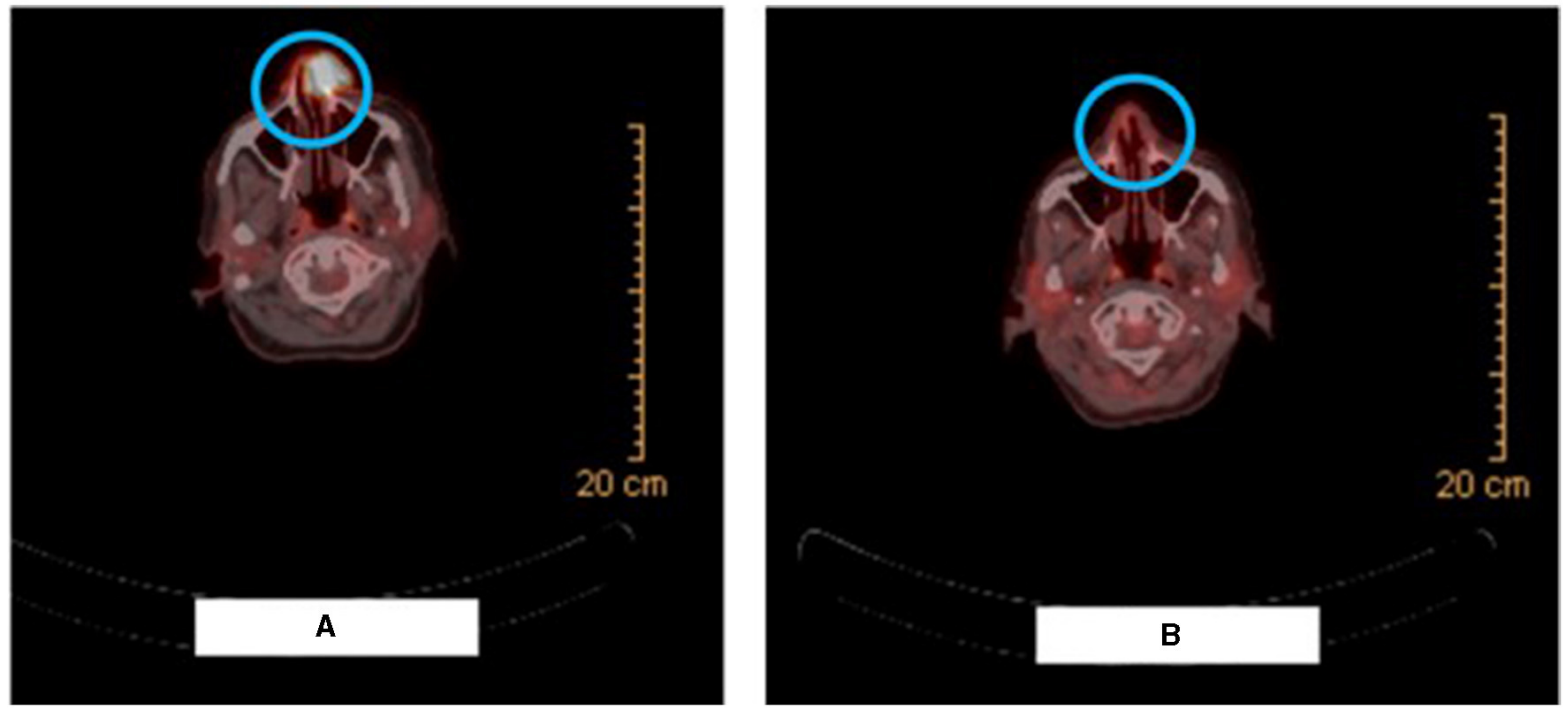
Radiologic picture on presentation vs after 2 cycles of neoadjuvant pembrolizumab. (A) Shows the PET-CT scan image demonstrating the radiotracer avid ulcerating soft tissue mass involving the left side of the nose causing nasal obstruction. (B) Shows the PET-CT scan image demonstrating resolution of the large radiotracer avid ulcerating soft tissue mass involving the left side of the nose and an interval decrease in activity, number, and size of the bilateral cervical lymph nodes.
